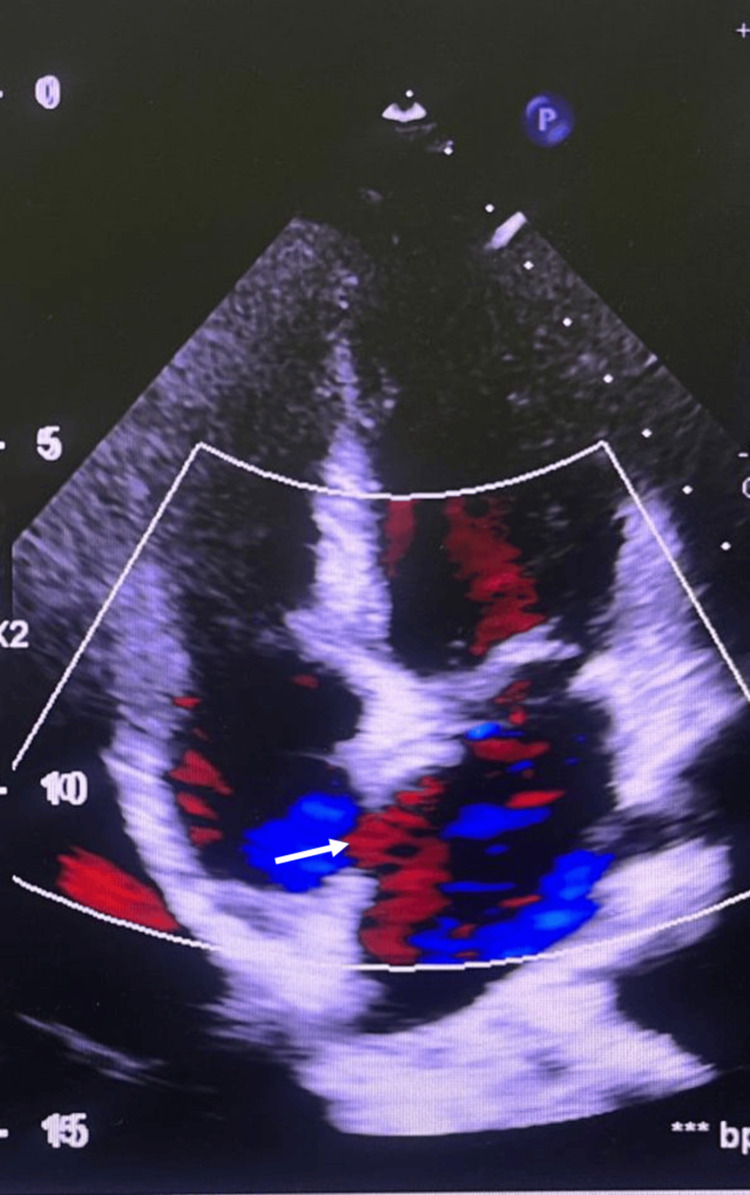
Color Doppler echocardiography (apical four-chamber view) of Case 4 showing a small ostium secundum atrial septal defect (ASD) measuring 8 mm with a minimal left-to-right shunt (white arrow) and no right-sided chamber enlargement, supporting conservative management.
